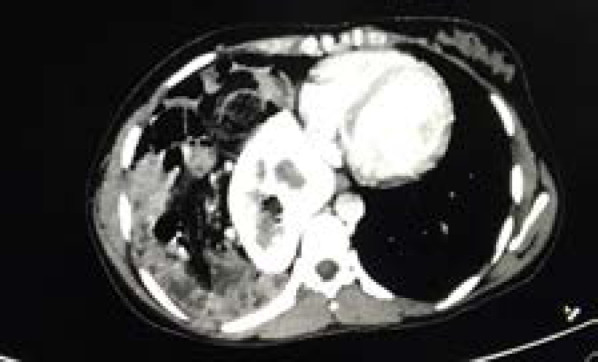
Axial view of CT showing the right kidney in the thoracic cavity near the pericardial region along with bowel loops.
